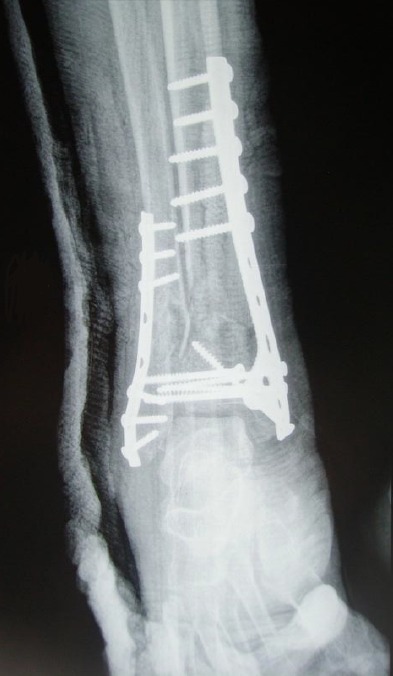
Radiographie de contrôle après à 2 mois du post opératoire n'objectivant pas de signes de sepsis sur matériel
